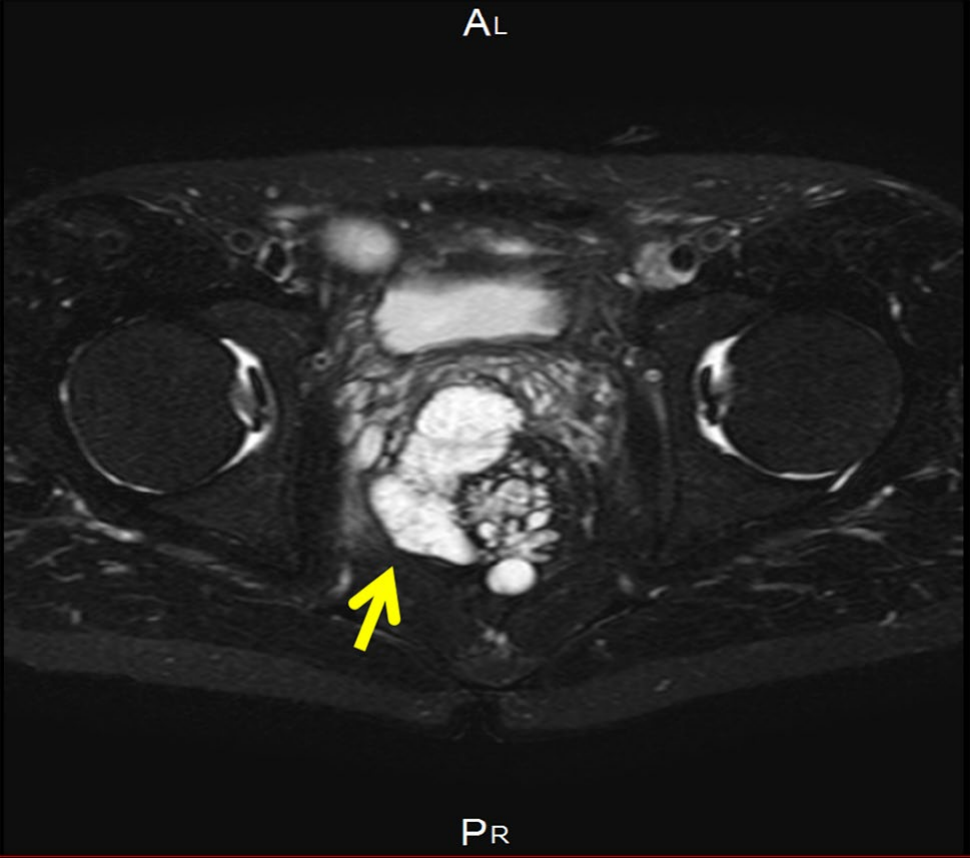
T2-weighted MRI findings of a representative case of mucinous cancer.* Arrow* indicates multilocular cyst
